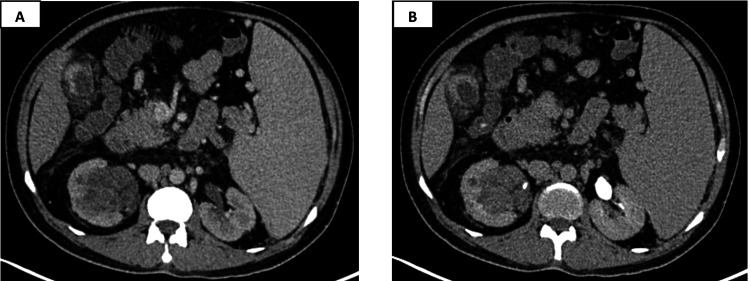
Contrast-enhanced CT images in venous (A) and delayed phases (B) showing a well-defined, solid, mildly enhancing lesion measuring 5.8 × 5.7 × 4.9 cm occupying the right renal pelvis causing a splaying of the collecting system.
